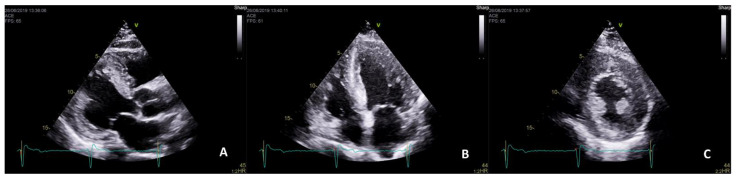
Left ventricular hypertrophy (LVH) secondary to Fabry disease (FD). Transthoracic echocardiogram of a 70-year-old male Fabry patient showing a severe symmetrical LVH with prominent papillary muscles in parasternal long-axis (A), four-chambers (B) and short-axis (C) views.
